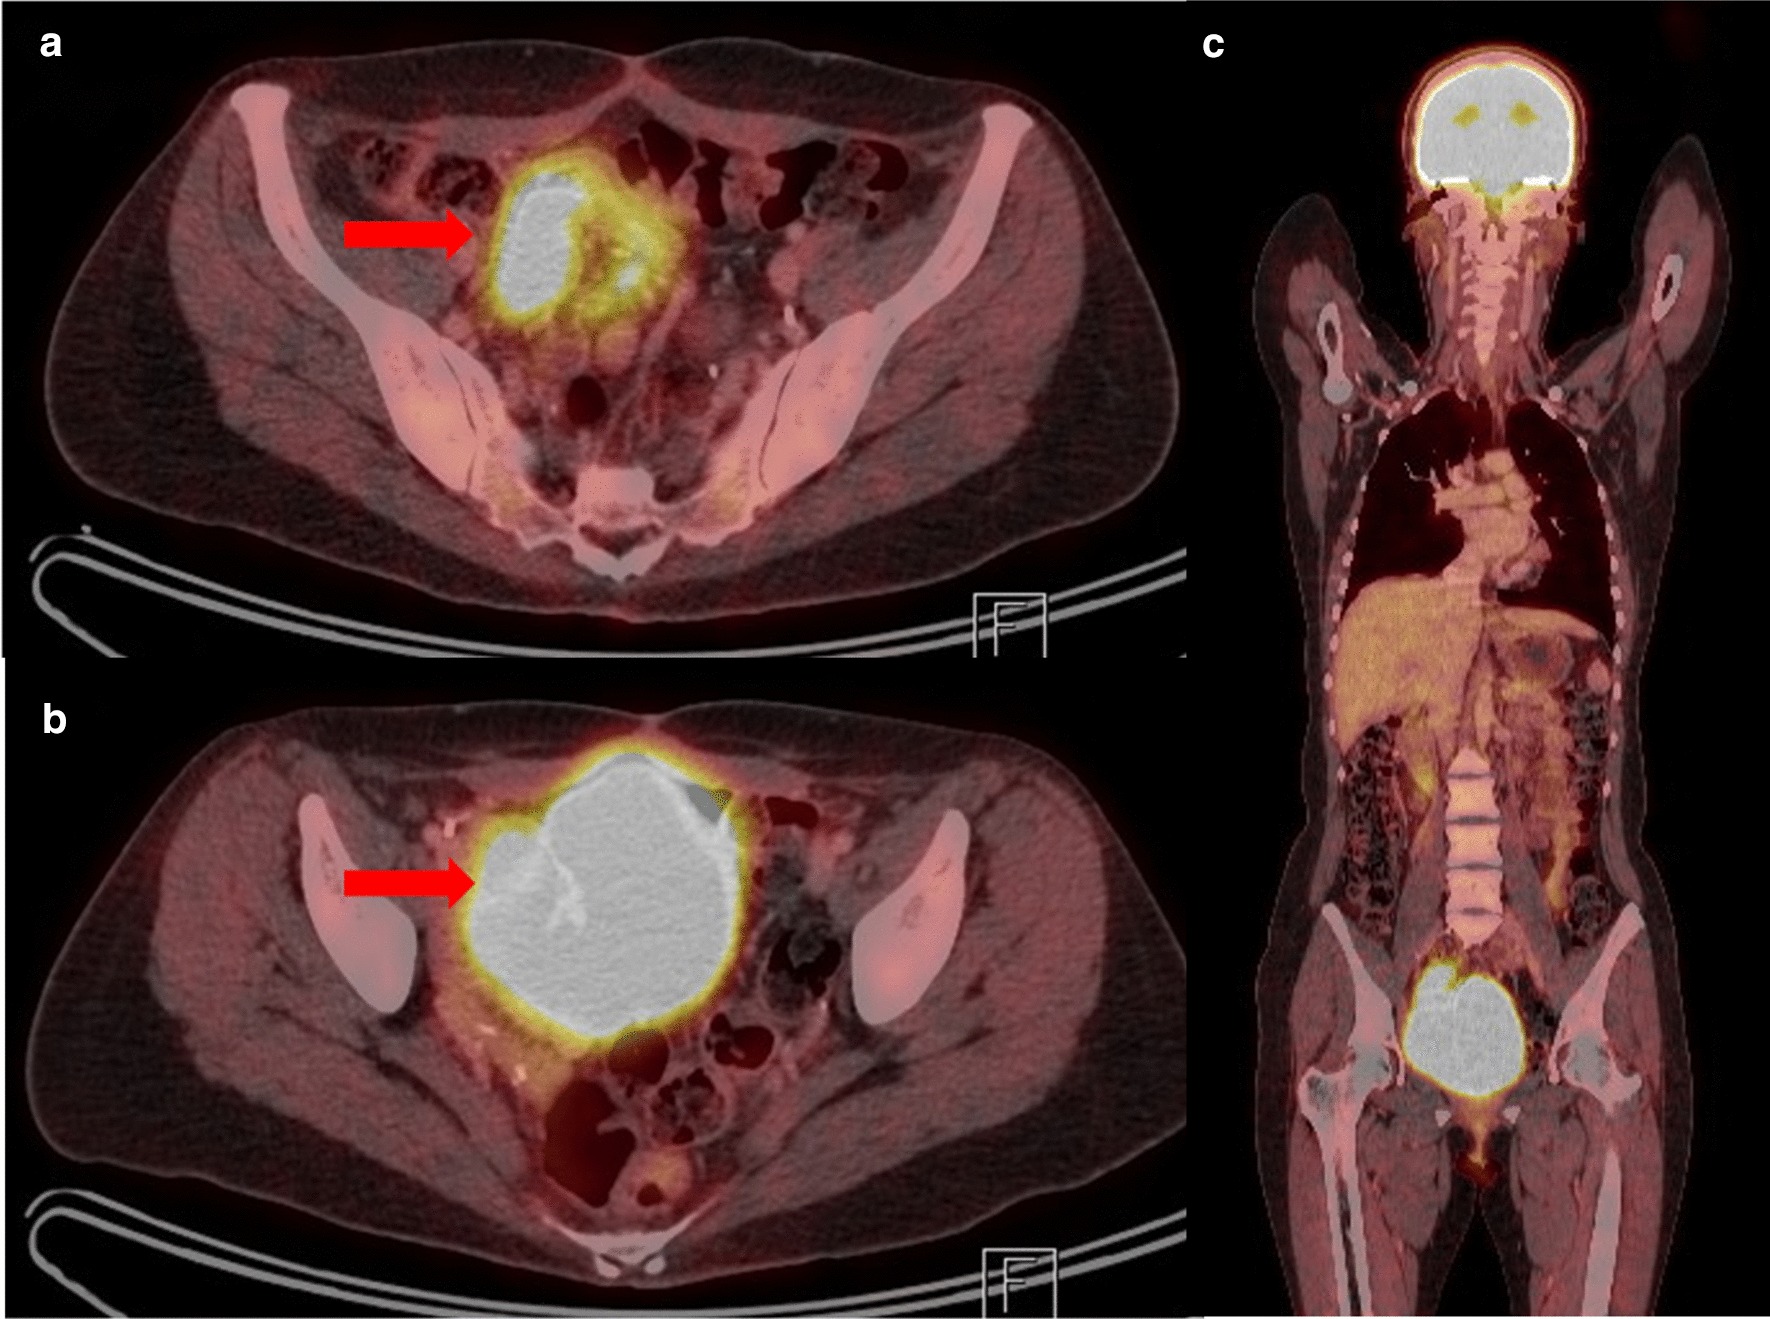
PET-CT with F18-FDG. There was no evidence of locoregional recurrence or distant metastasis after 18 months of surveillance. a Axial view of chimney (arrow). b axial view of neobladder (arrow). c Coronal view
